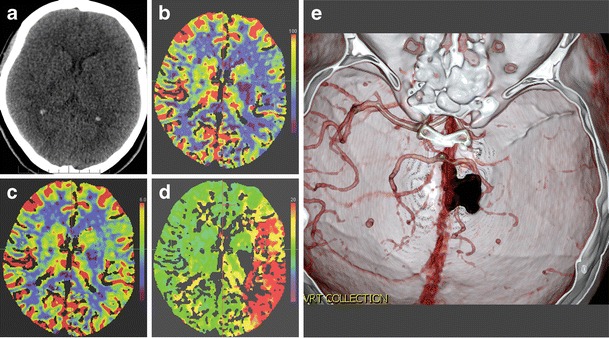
Acute ischemic stroke due to obliteration of the left ICA and MCA. a Non-contrast CT is practically normal, with no hypodense areas and no mass effect present. b CBF map shows hypoperfusion (colder colours) in the region of the left MCA. c CBV map with only subtle differences between left and right hemispheres. d TTP map displays a significant delay in the region of the left MCA due to collateral flow. e CT angiography displays obliteration of the left MCA. ACI, internal carotid artery; MCA, middle cerebral artery; CBF, cerebral blood flow; CBV, cerebral blood volume; TTP, time-to-peak
